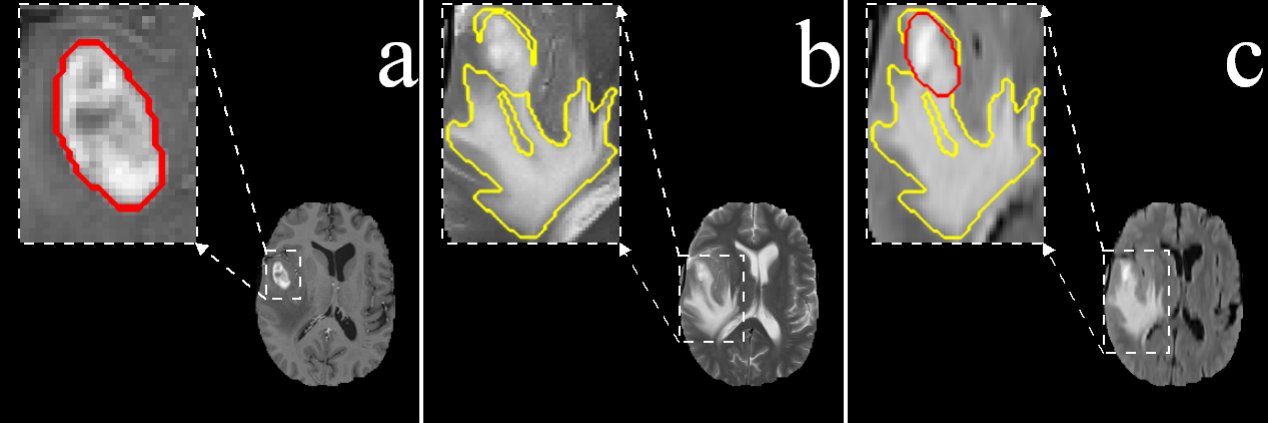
Primary MRI sequences of the brain. (a) *T*1*C* exhibiting enhanced tumor structure and boundary (Red), (b) *T*2 associated with edema or swelling (Yellow), and (c) *FLAIR* demonstrating both the edema (Yellow) and enhanced solid core (Red).
